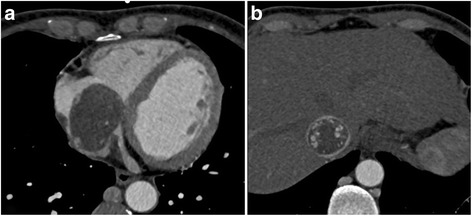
Cardiac CT scan showing a hypodense mass occupying the *right* atrium (a) and the inferior vena cava in toto (b)
